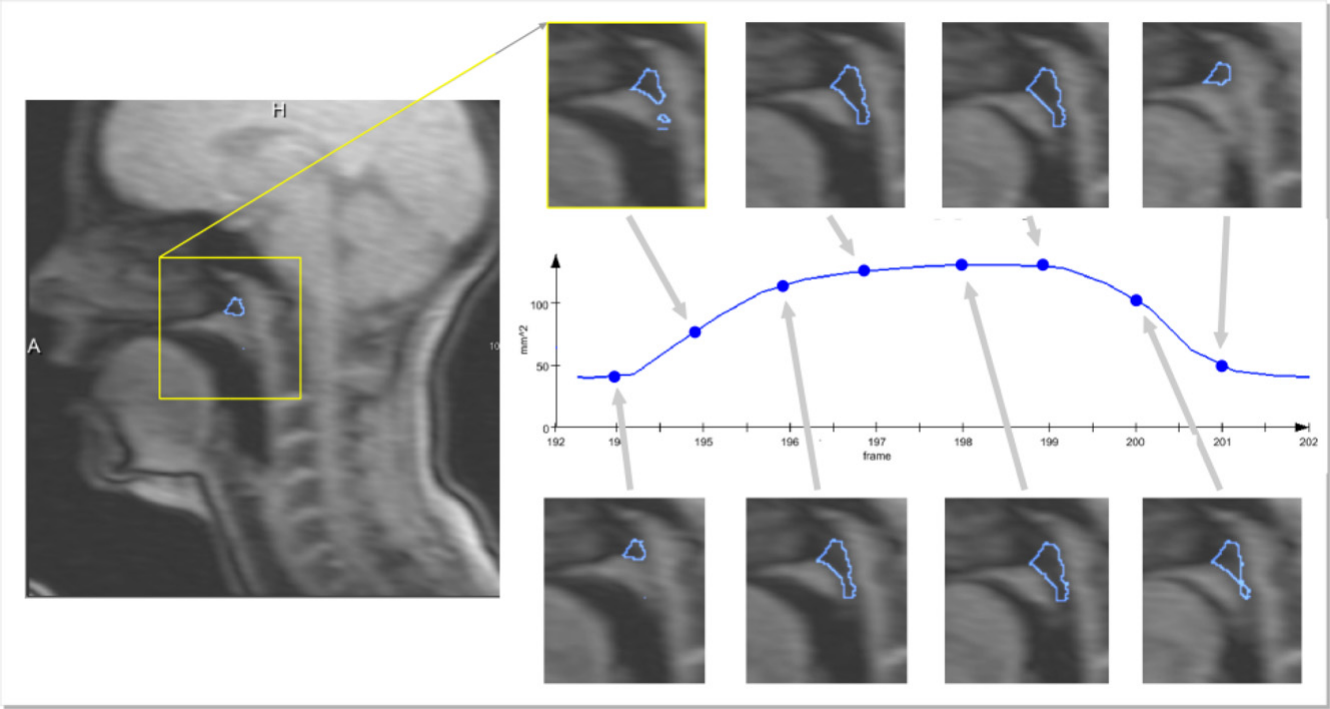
Mid-sagittal RT-MRI images of the vocal tract for several velum positions, over time, showing evolution from a raised velum, to a lowered velum and back to initial conditions. The presented curve, used for analysis, was derived from the images.
